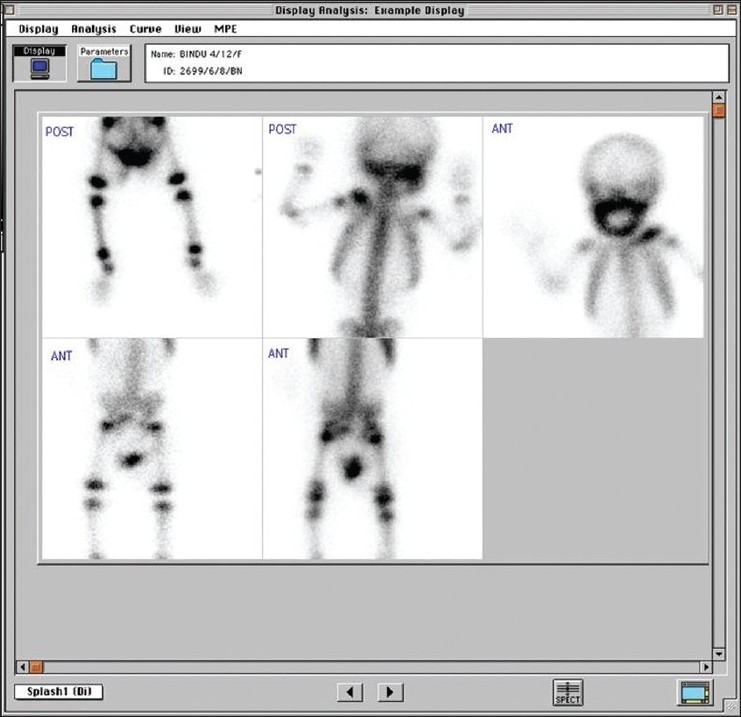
99m Tc MDP skeletal scintigraphy showing diffusely increased uptake in both mandibles, with similar uptake in both clavicles, coinciding with the radiological lesions
